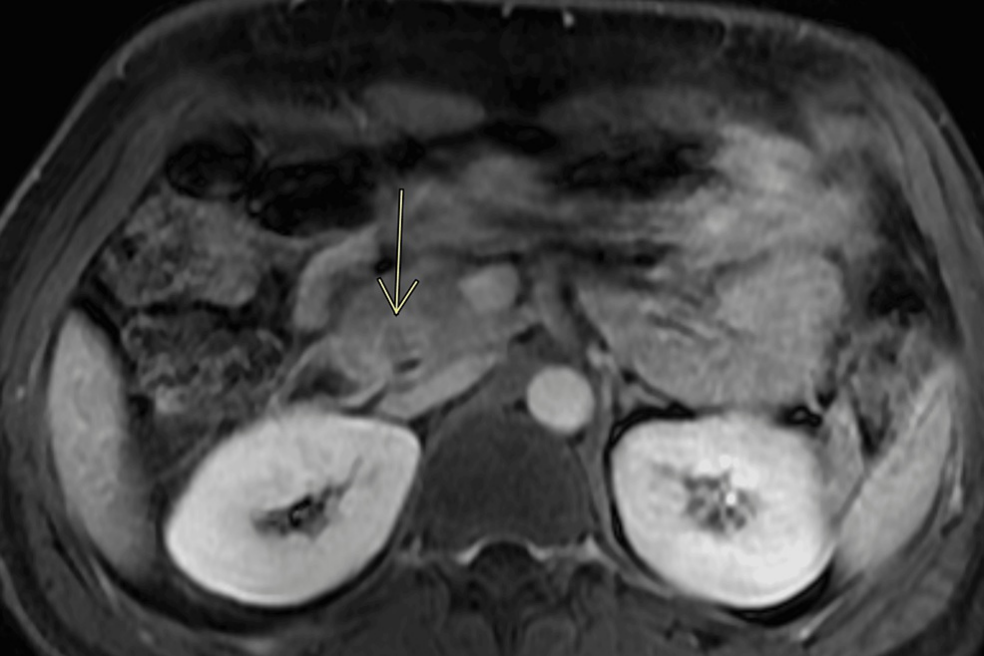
Pancreatic MRI The arrow shows a mass in the pancreatic head MRI: magnetic resonance imaging
